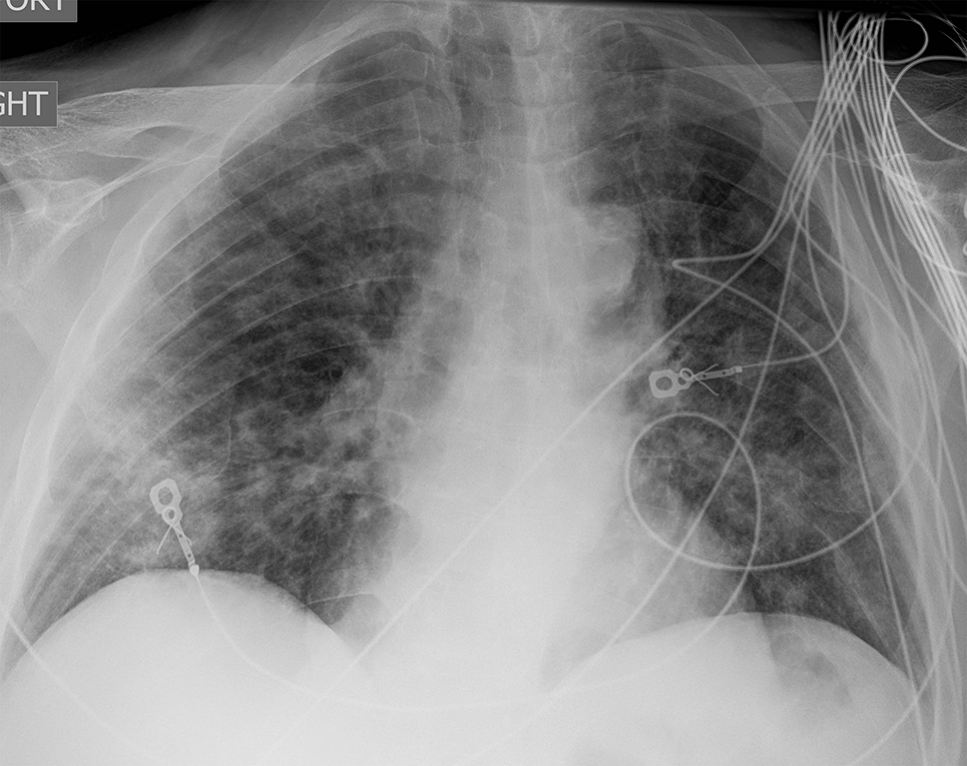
Chest X-Ray on admission demonstrating bilateral heterogeneous pulmonary opacities
